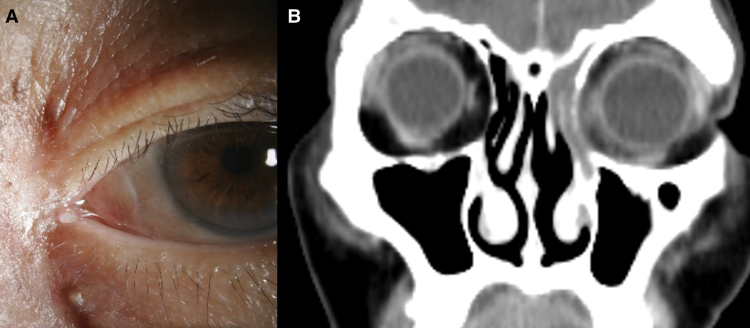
Clinical and radiologic findings 3 months after the second cycle of chemotherapy. (A) Clinical photograph showing complete healing of the left medial canthal skin defect. (B) Follow-up facial computed tomography showing remission of the previously noted soft tissue mass involving the left ethmoid sinus and medial orbit.
